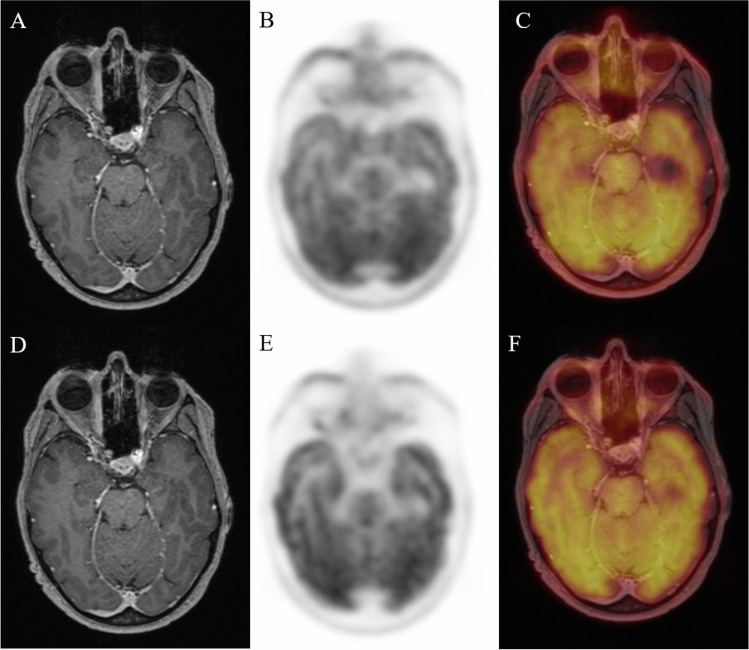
Transaxial MRI (panels A, D), PET (panels B, E), and fused slices of a 39-year-old patient with mild GO reveal slight hypertrophy of orbital tissue and faint ^18^F-FDG uptake, both on early (panels A–C) and late static images (panels D–F)
